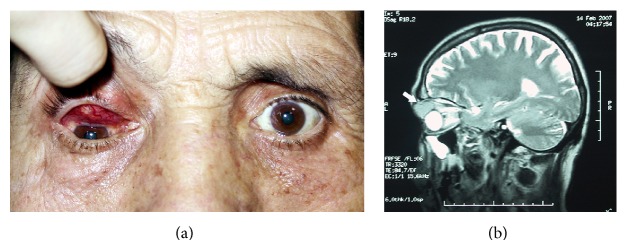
(a) Clinical appearance of the conjunctival mass lesion. Note that the globe is significantly displaced inferiorly. (b) Magnetic resonance imaging of the conjunctival mass lesion (arrow).
